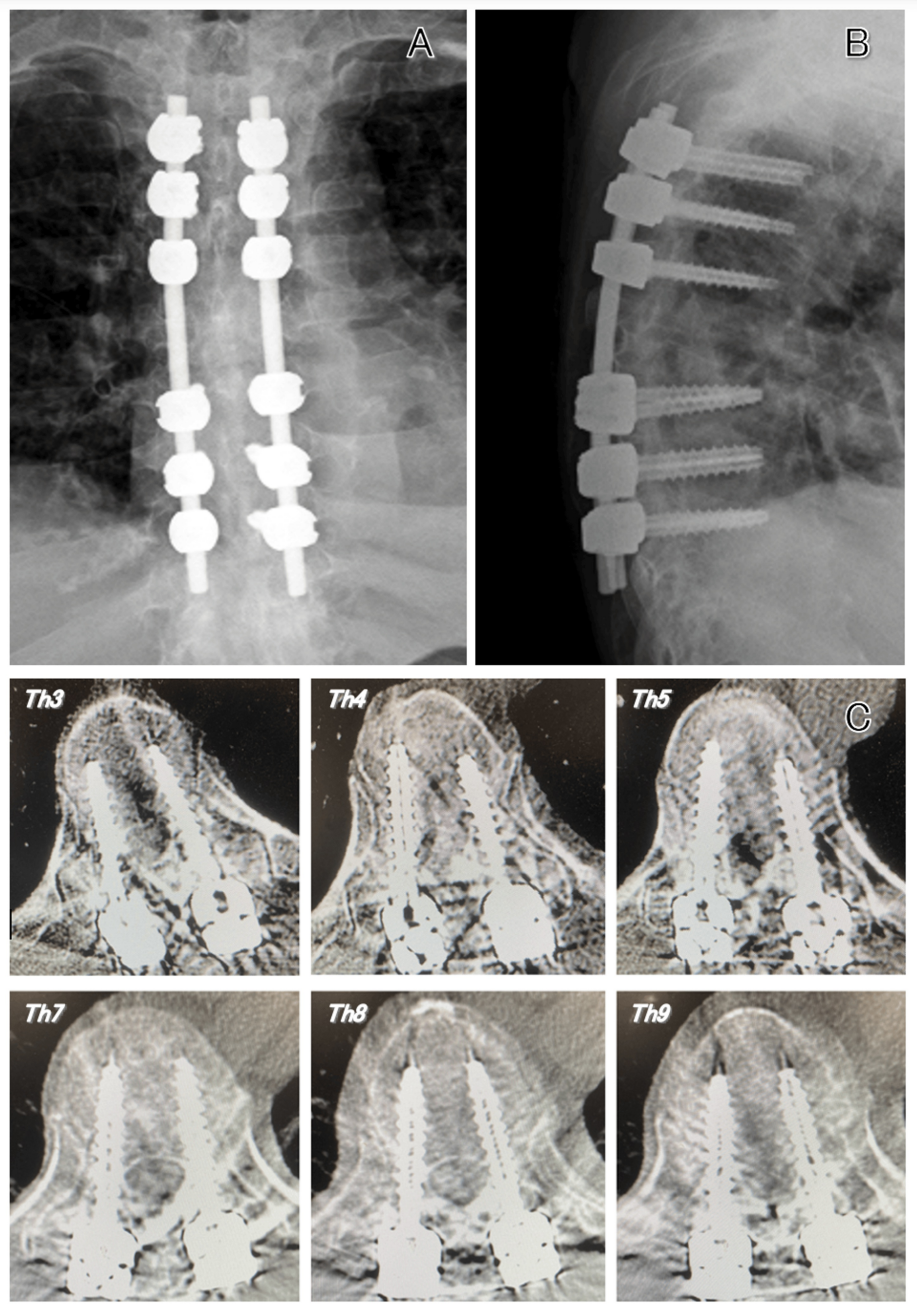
Postoperative images. (A, B) X-ray fluoroscopy shows that the rods are almost parallel on both sides, and the screws are long enough to be inserted. (C) Computed tomography scan shows none of the screws deviated outside the pedicles.
